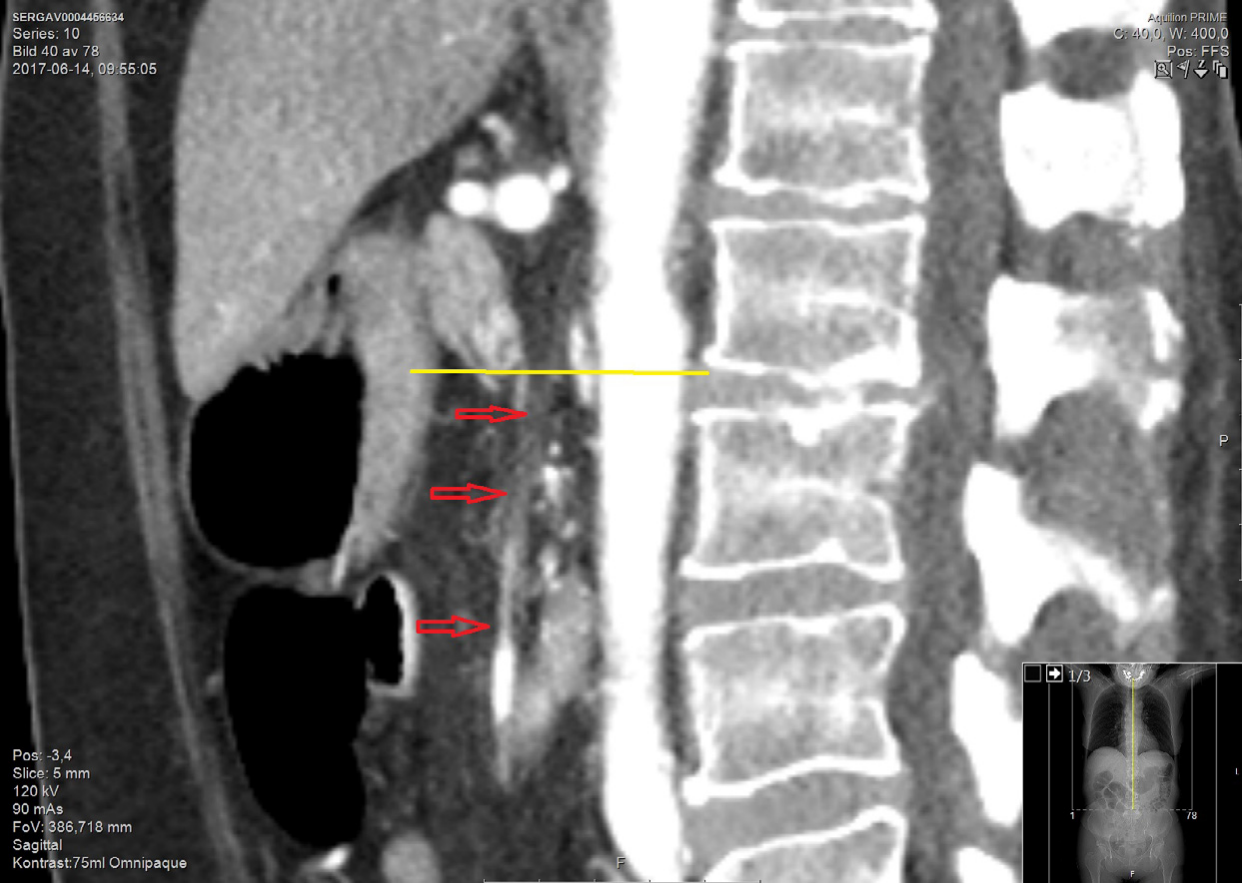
Abdominal computed tomography scan demonstrating the total occlusion of superior mesenteric artery (sagittal).
